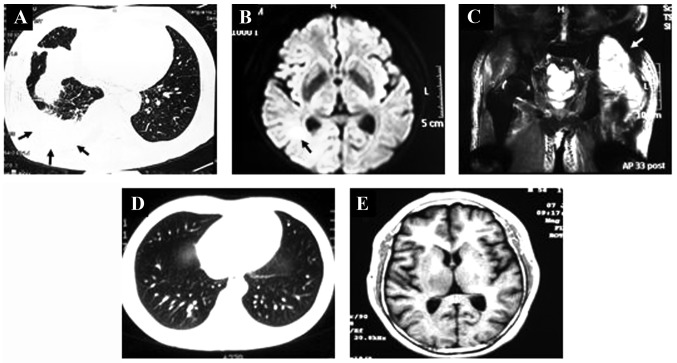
MRI and CT scans results prior to and following treatment with trimethoprim-sulfamethoxazole. (A) CT scan shows abscesses in the right lung prior to treatment. MRI scans reveal (B) an abscess in the right brain, as well as (C) a large abscess in the left gluteal region prior to treatment. (D) CT scan shows that the abscess in the right lung disappeared following treatment. (E) MRI shows that the abscess in the right brain disappeared following treatment. MRI, magnetic resonance imaging; CT, computed tomography.
